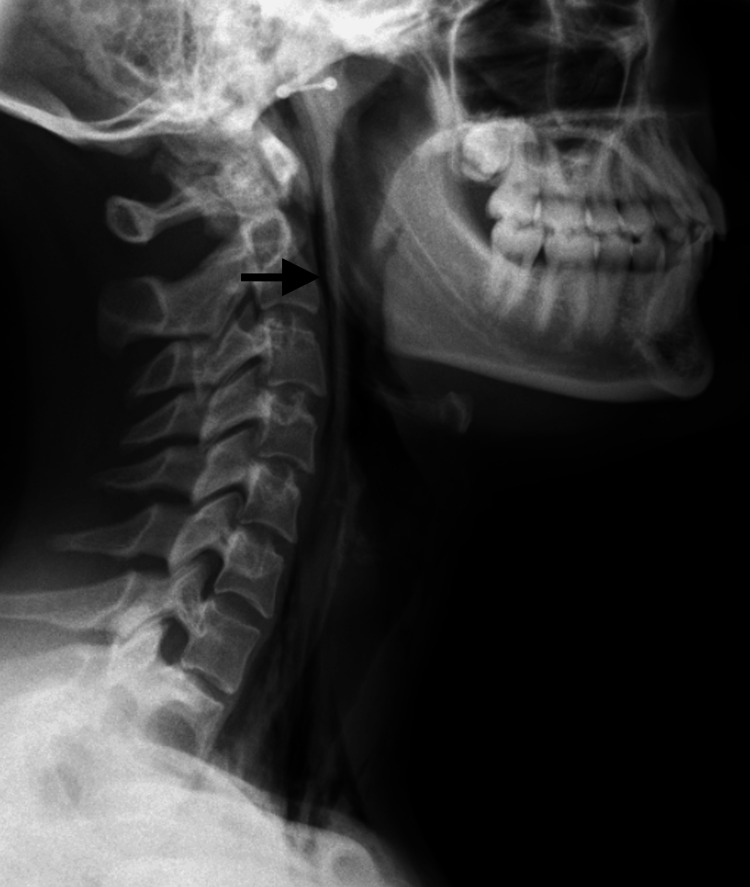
Lateral neck X-ray demonstrating retropharyngeal emphysema
